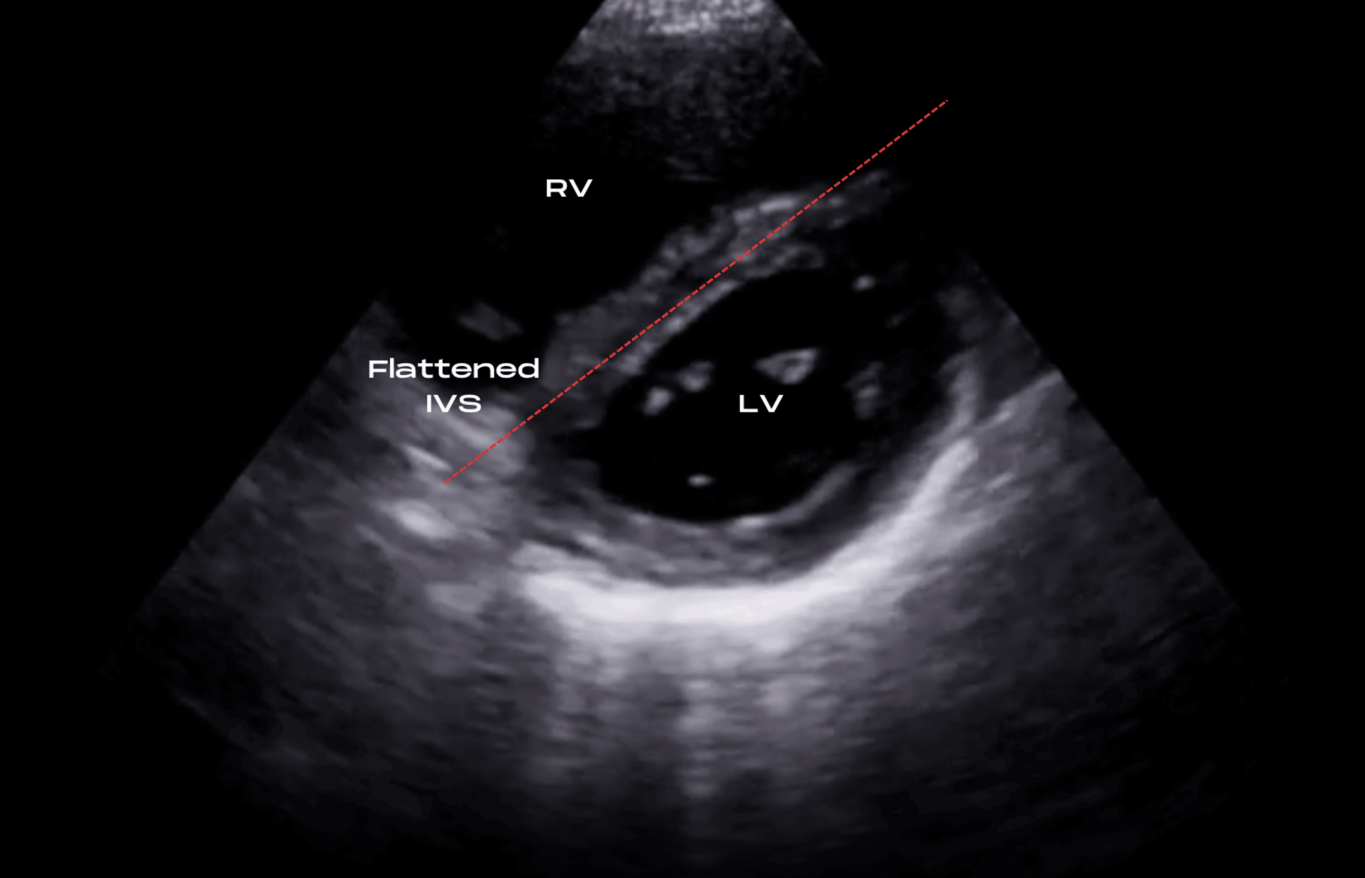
PSAX view with flattening of the interventricular septum, also known as the 'D sign,' indicative of raised RV pressure. PSAX: Parasternal Short Axis View; IVS: Interventricular Septum; RV: Right Ventricle; LV: Left Ventricle.
